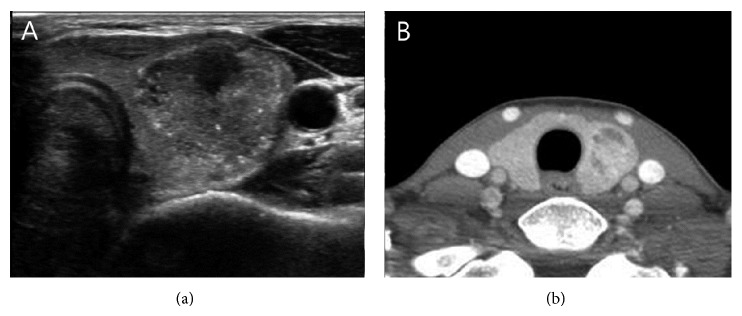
44-year-old woman with papillary thyroid carcinoma without extrathyroidal extension. On contrast to the US finding which shows the tumor closely attached to the capsule, CT findings revealed the complete parenchymal envelop around the tumor.
